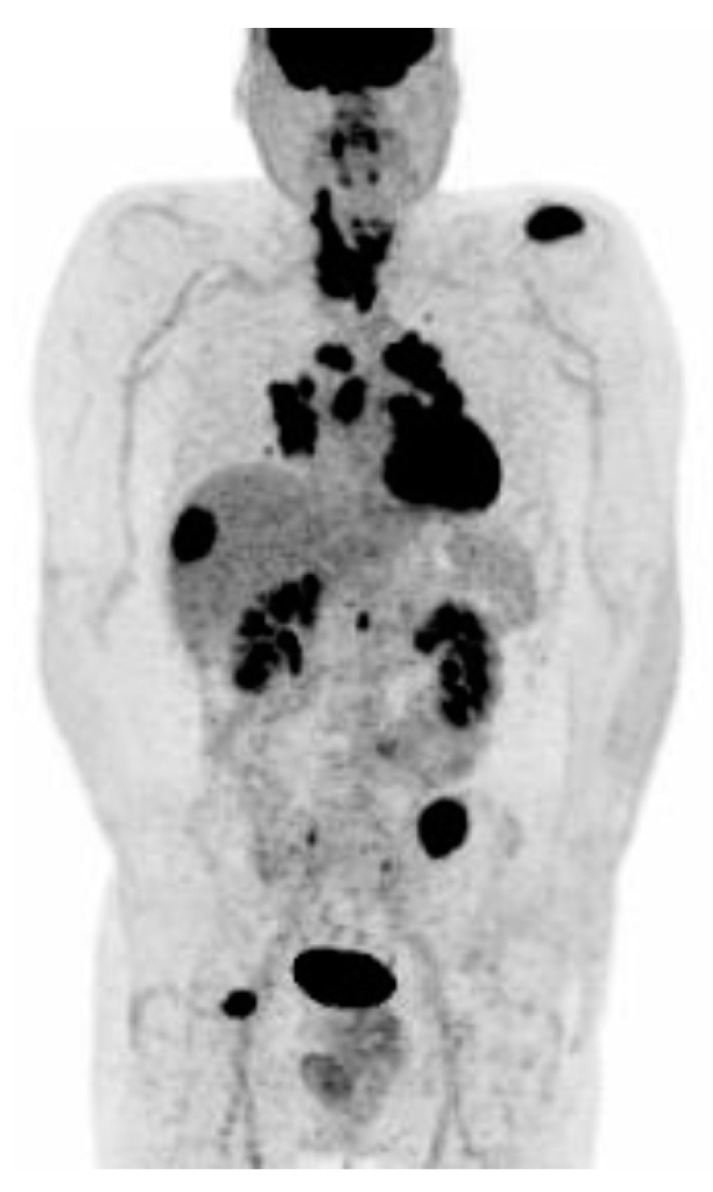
Advanced radioiodine-refractory and [^18^F]FDG-avid metastatic thyroid carcinoma involving the thyroid “in toto” with multiple metastases (lymph nodes, lung, liver, kidney, and bone).
